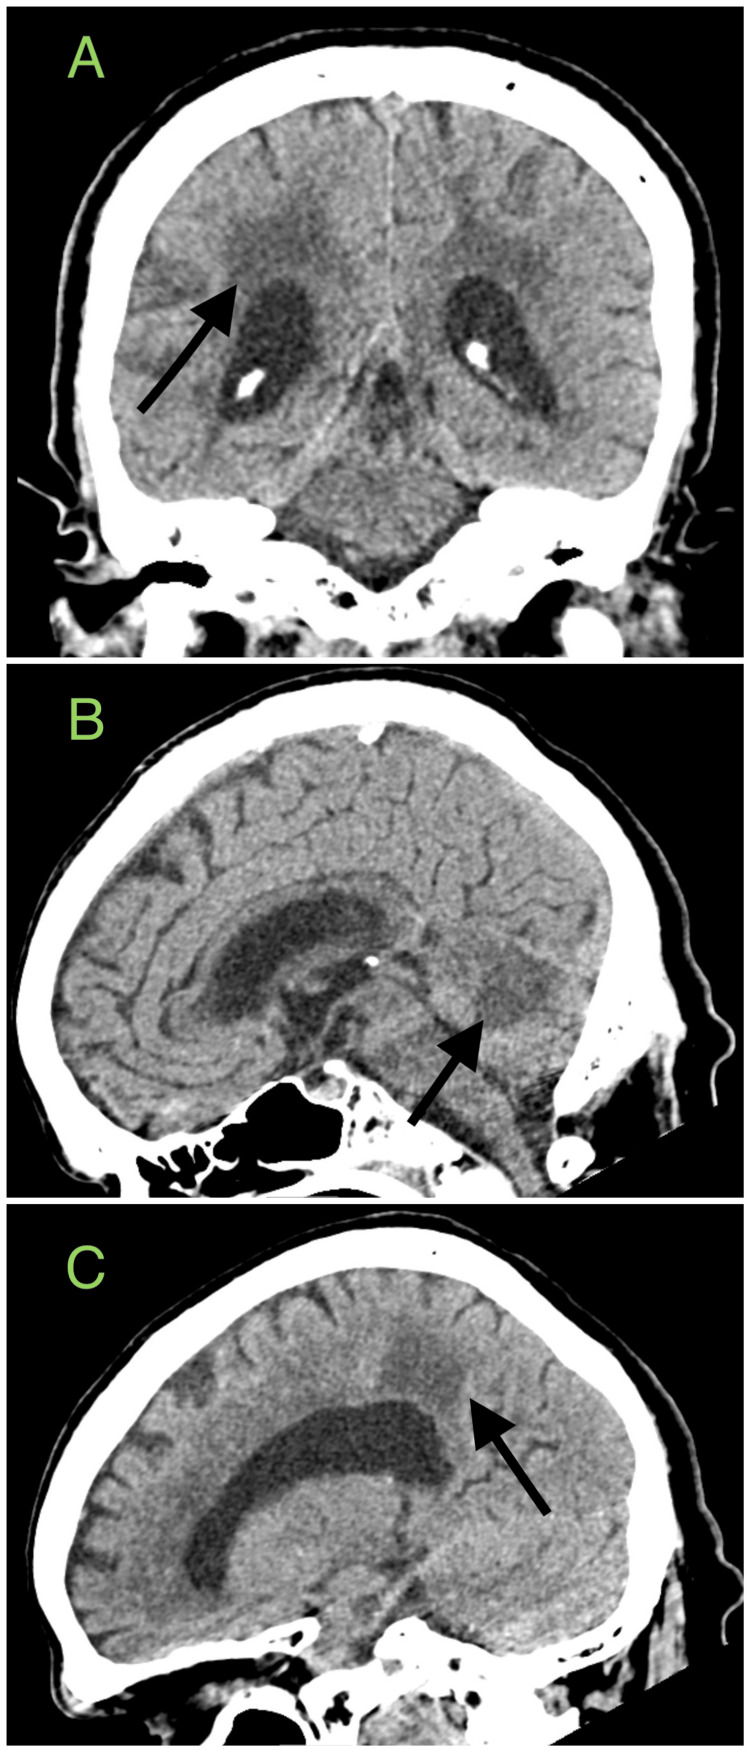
Brain CT without contrast Black arrows in images A, B, and C show hypoattenuation representing acute infarction.
